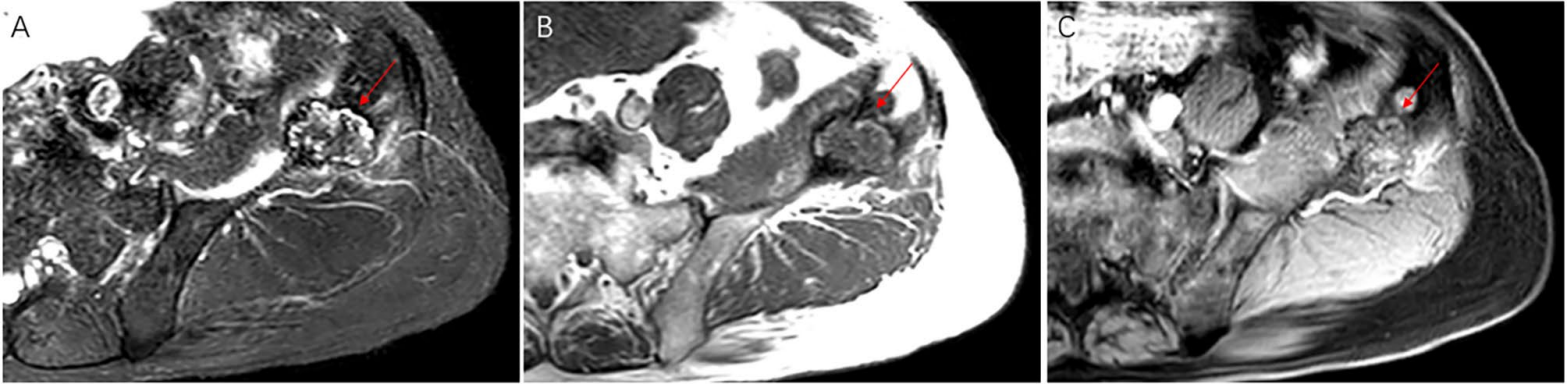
The lesion showed heterogeneous signal on the fat-suppressed sequences of T2-weighted MR images and T1-weighted MR images, with central low signal and peripheral linear high signal (A-B, arrow). The size is about 30 mm×26 mm. After injection of the contrast agent (Gd-DTPA), the lesion showed inhomogeneous enhancement (C, arrow)
